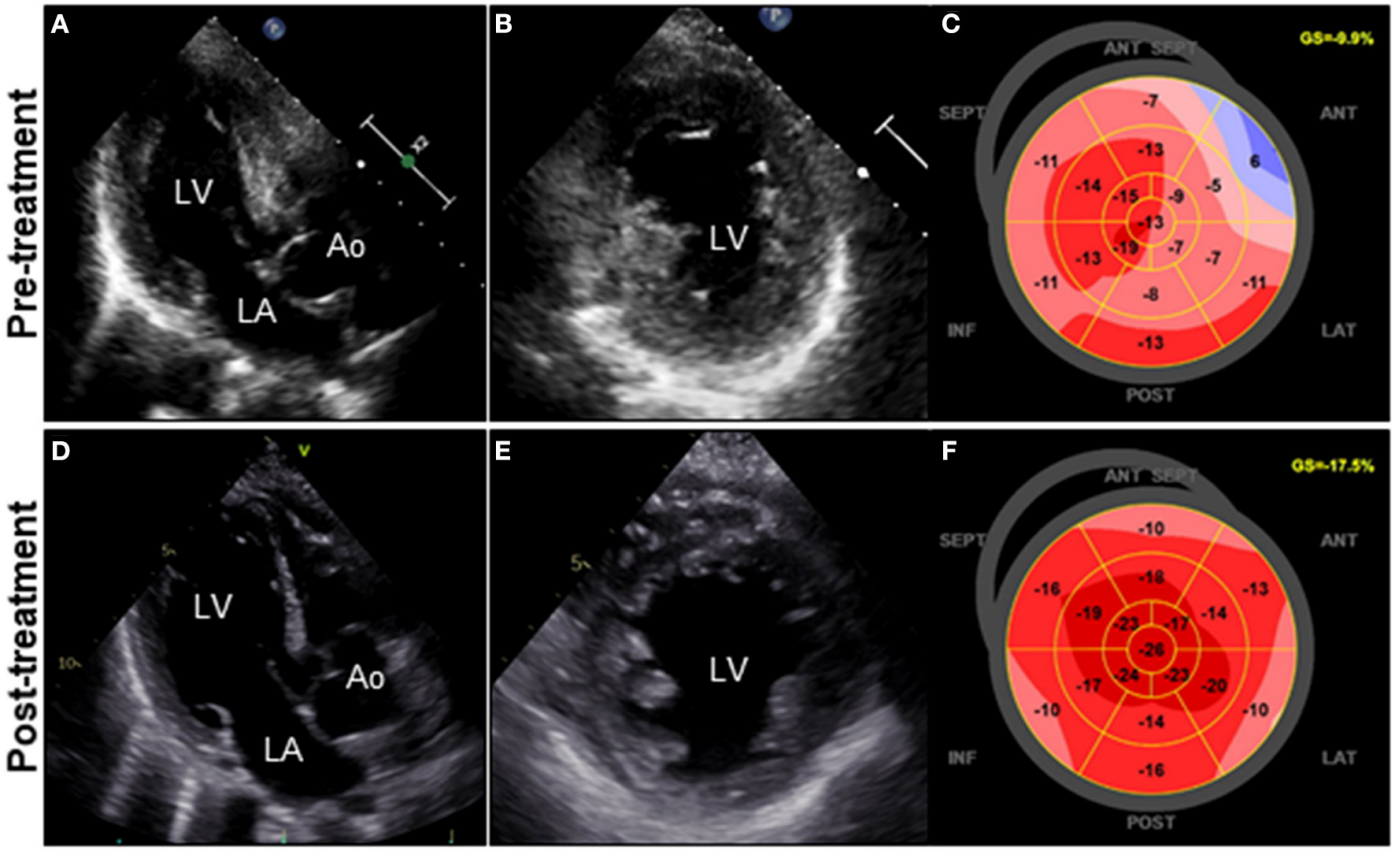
Clinical effects of corticosteroid treatment on TTE. TTE on admission reveals diffuse and symmetrical LV wall thickening (11 mm), decreased cavity size, reduced ventricular function, and GLS values of the LV (LVDd, 44 mm; LVEF, 43%; and GLS, −9.9%; respectively) (A–C). Follow-up TTE on day 21 after corticosteroid therapy reveals a significant decrease in LV wall thickness (8 mm) with concomitant improvement in cavity size, ventricular function, and GLS values of the LV (LVDd, 48 mm; LVEF, 50%; and GLS, −17.5%; respectively) (D–F). Ao, aorta; GLS, global longitudinal strain; LA, left atrium; LV, left ventricle; LVDd, left ventricular end-diastolic diameter; LVEF, left ventricular ejection fraction; TTE, transthoracic echocardiography.
